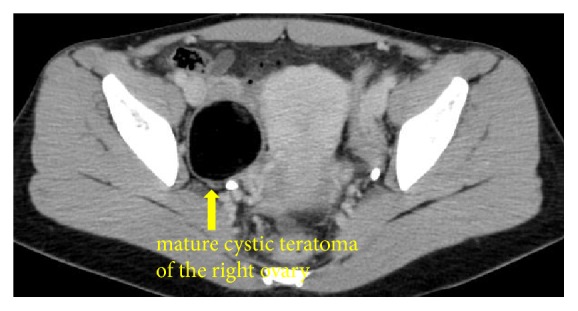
Contrast-enhanced computed tomography image of the pelvic cavity.
